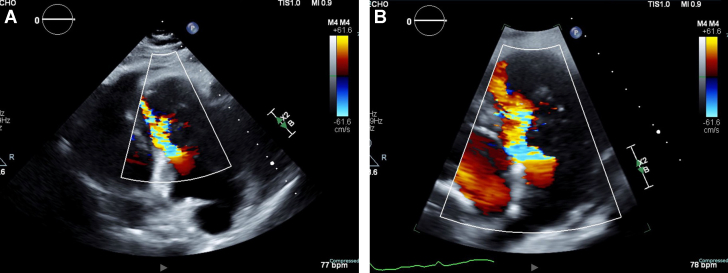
Color Doppler on Transthoracic Echocardiography Demonstrating Turbulent Flow Within the Right Ventricle and Right Ventricular Outflow Tract The aliasing pattern (multicolor flow) suggests increased velocity due to RVOT obstruction caused by the large right ventricular mass. Findings indicate hemodynamically significant obstruction due to RV mass.
